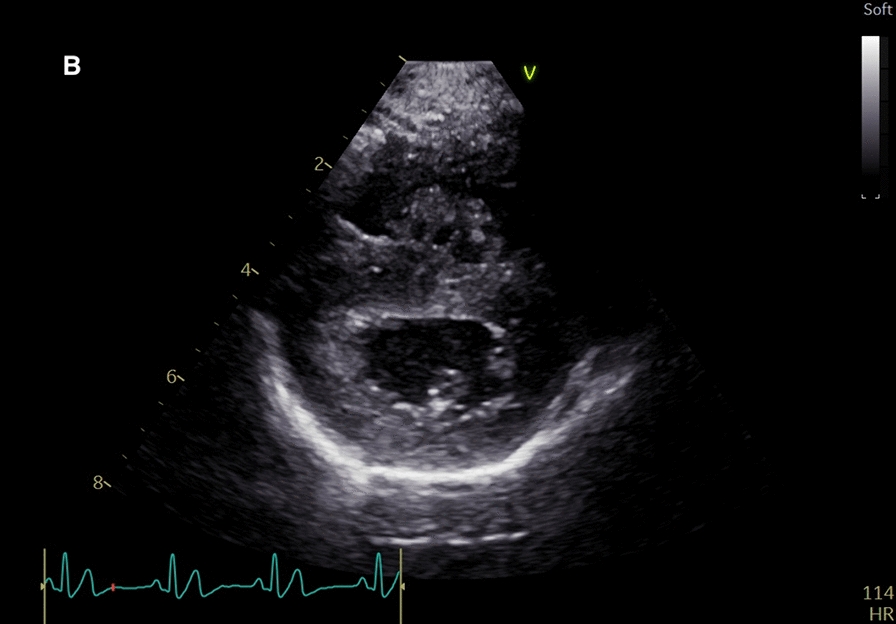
Echocardiography performed pre-treatment (B) from the right parasternal long axis four chamber view showing mild right chamber dilation, and from the short axis view showing mild interventricular septal flattening
